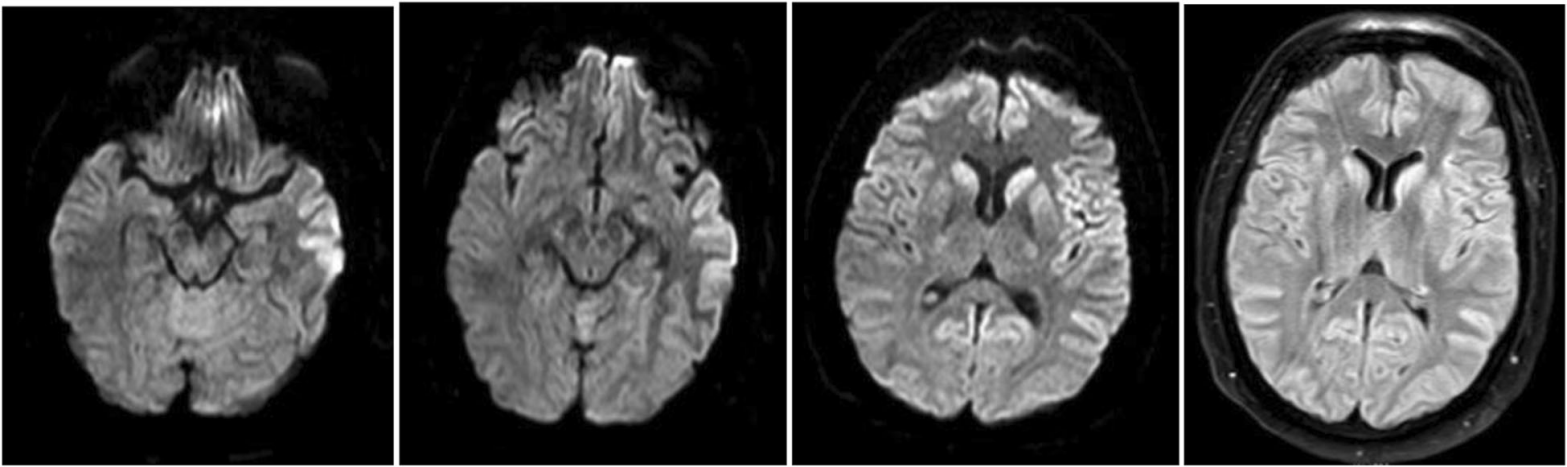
Second brain MRI showing increased DWI and T2 (FLAIR) signal in basal ganglia and cerebral cortex (cortical ribbon sign)
